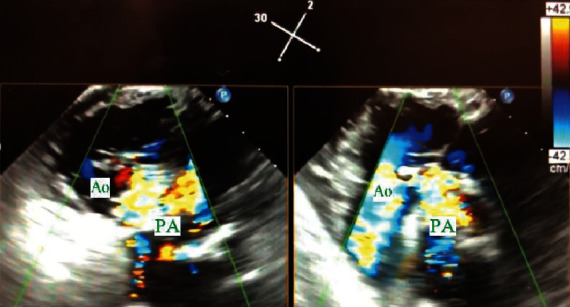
Transesophageal echocardiogram showing aortopulmonary fistula. View: color Doppler, long axis view—upper esophageal. AO: ascending aorta; PA: pulmonary artery.
